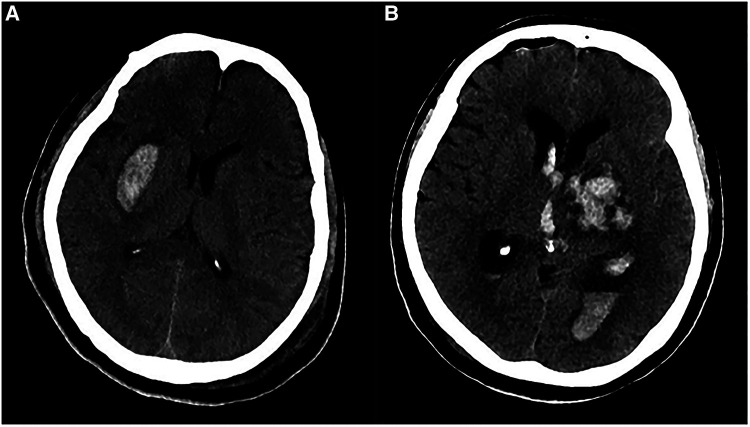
Postoperative imaging of patients with basal ganglia hemorrhage. (A) Postoperative CT in a patient with left TN showed a right external capsule hemorrhage. (B) Postoperative CT in a patient with right TN showed a left basal ganglia hemorrhage which ruptured to ventricles.
